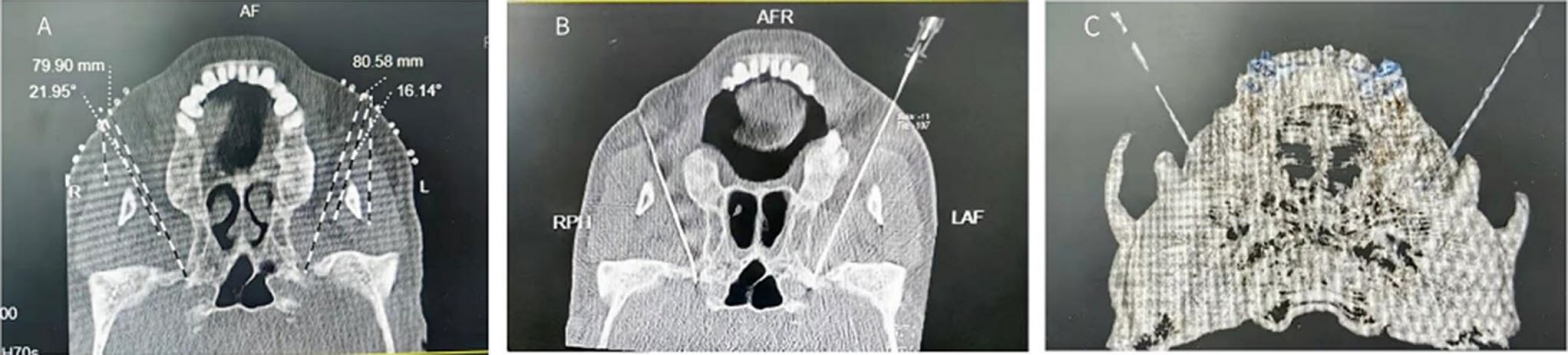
Radiofrequency ablation of mandibular branch of trigeminal nerve by bilateral foramen ovale puncture under CT guidance. (A) The puncture path design of bilateral foramen ovale. The puncture depth and angle of the patient’s left and right sides are 7.99 cm, 8.05 cm, 16.14° and 21.95° respectively. (B) the successful image of bilateral foramen ovale puncture, the puncture tip is flat on the inner orifice of foramen ovale and does not enter the skull; (C) CT three-dimensional reconstruction image after successful puncture of bilateral foramen ovale, with bilateral RF needle points located in the foramen ovale
